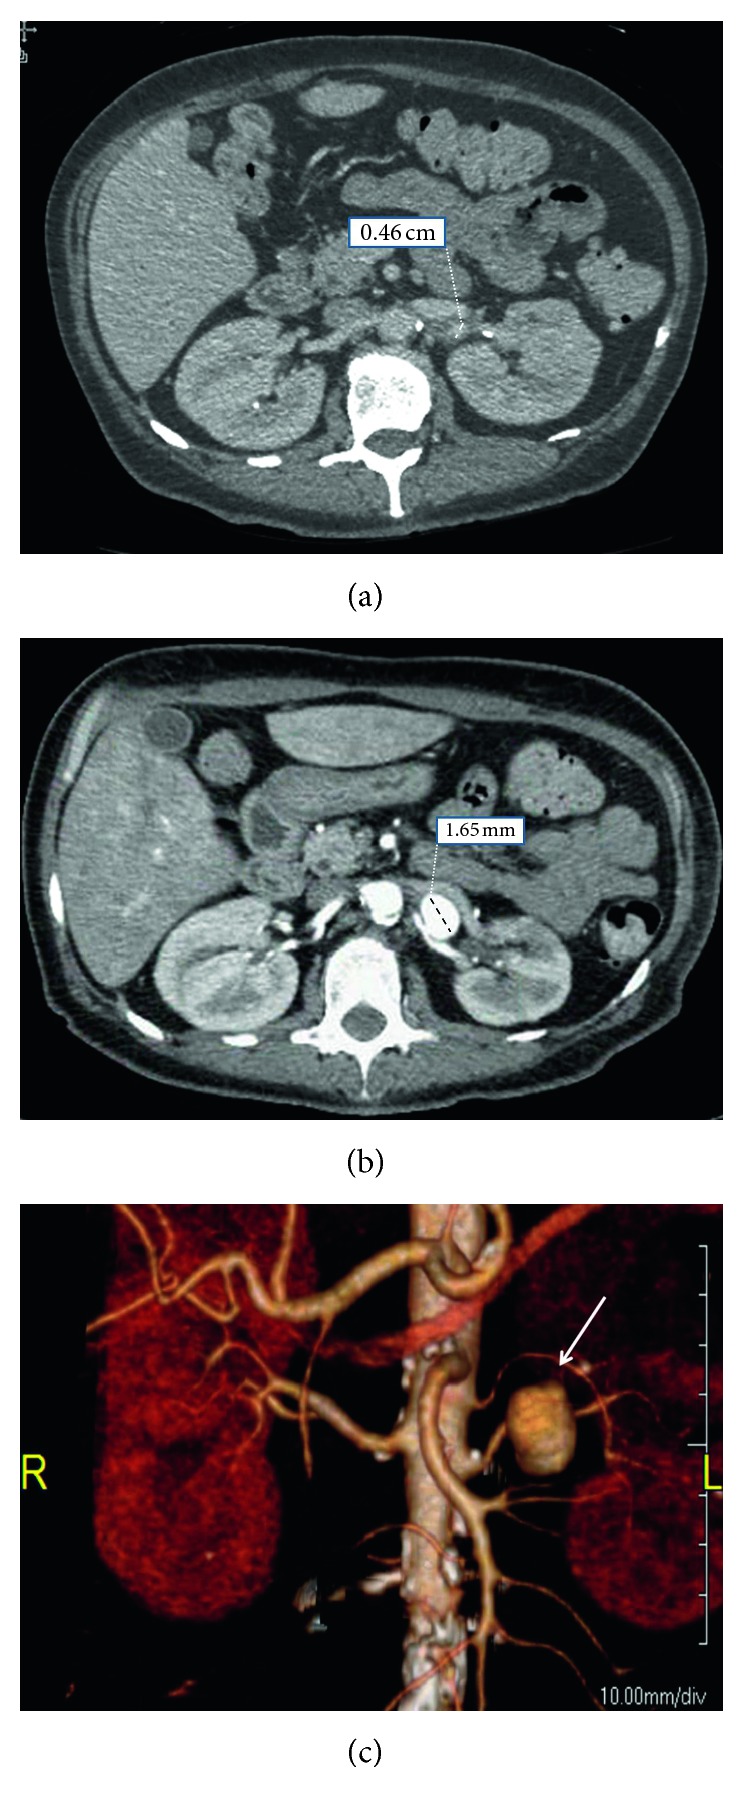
CT axial images demonstrating (a) normal caliber left renal artery 3 weeks prior to presentation, (b) 1.65 cm left renal artery aneurysms, and (c) 3D reconstruction of left renal artery aneurysm (arrow).
